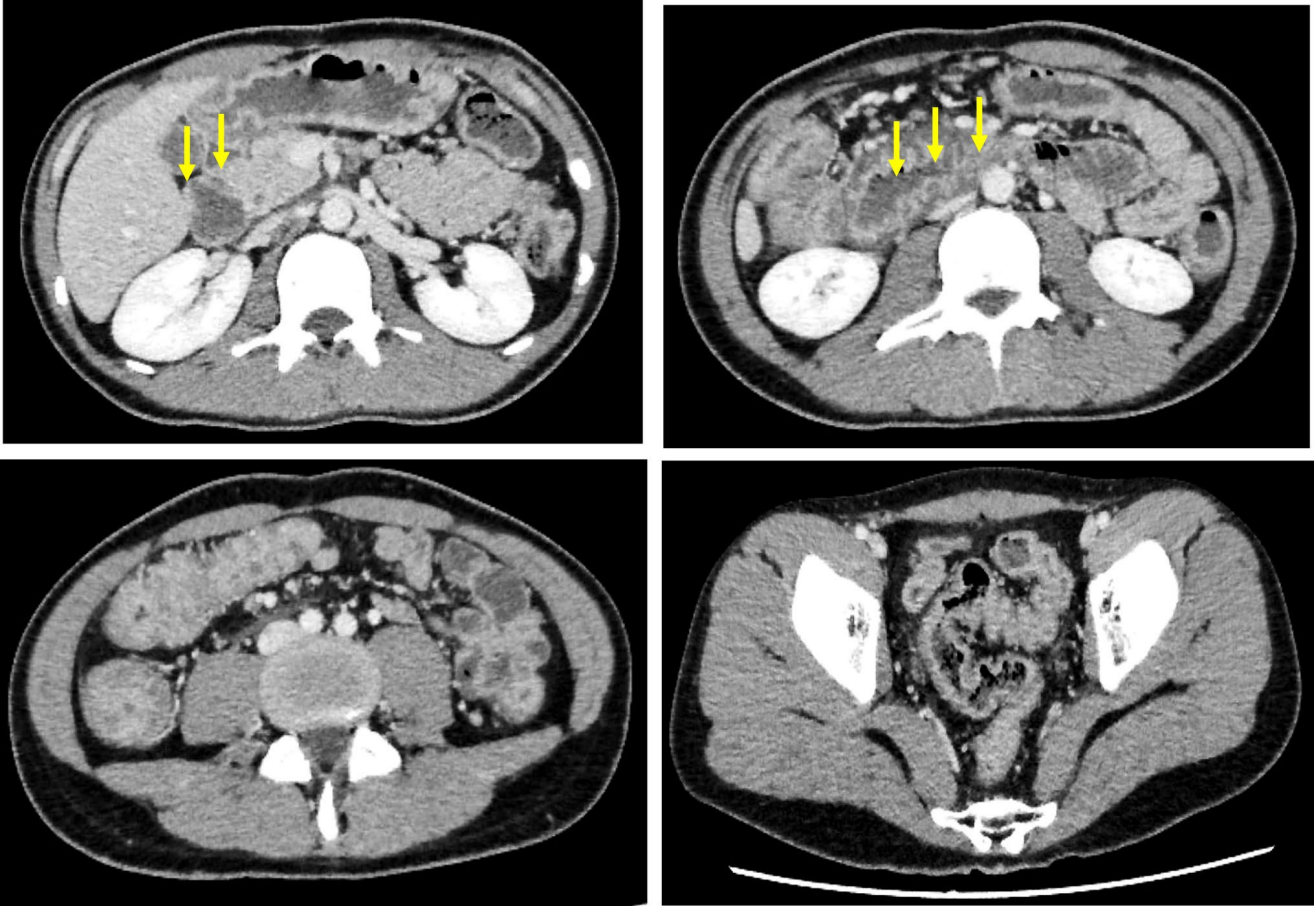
Contrast-enhanced computed tomography of the abdomen.The entire colon and the duodenum showed enhanced marked wall thickening (arrow)
